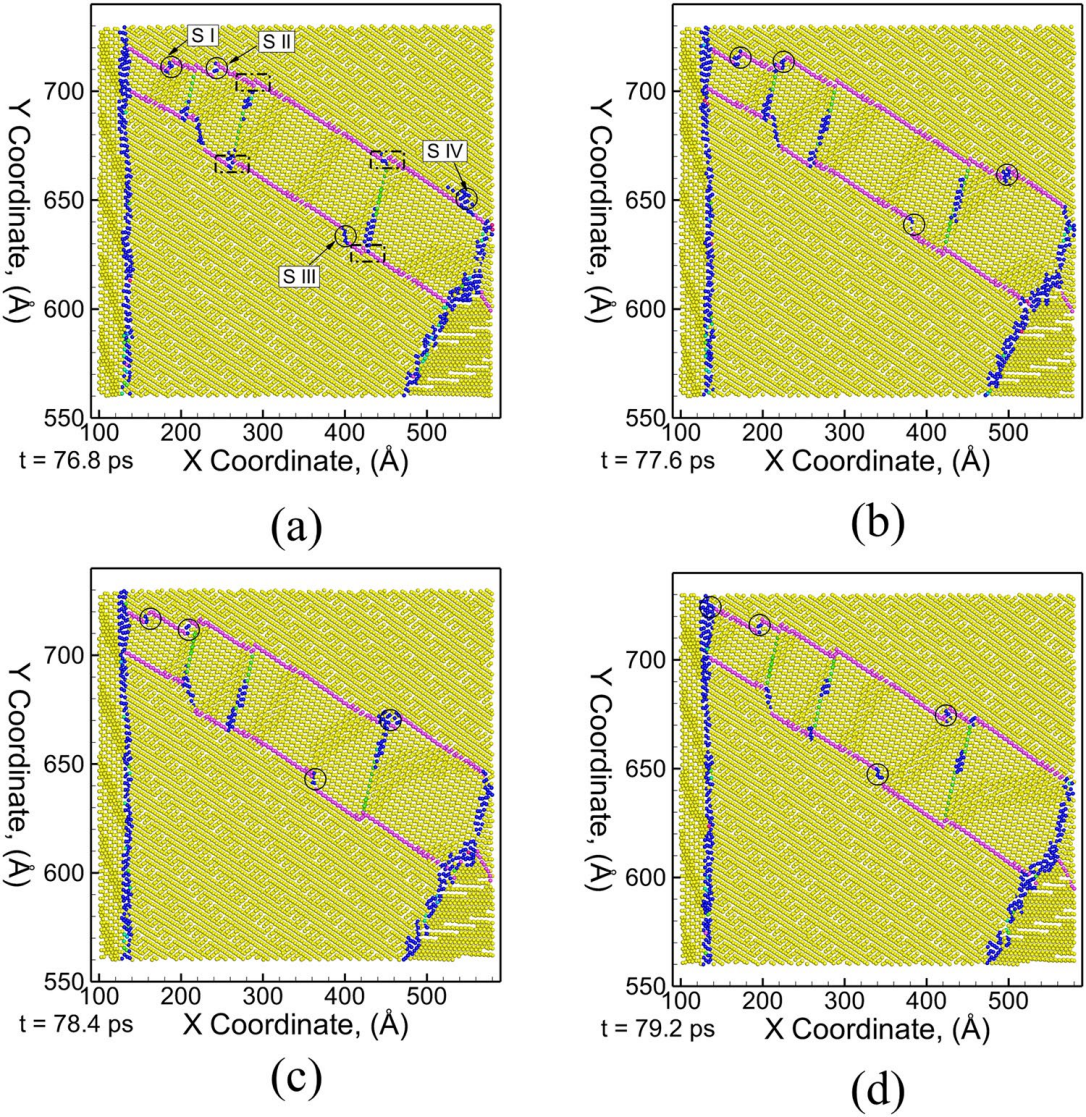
Microstructural snapshots depicting the growth of {$$10\bar{1}1$$} CT by movement of steps along the twin boundary during uniaxial tensile stress loading at a strain rate of 10^9^ s^−1^ at a time (strain) of (a) 76.8 ps (7.68%), (b) 77.6 ps (7.76%), (c) 78.4 ps (7.84%) and (d) 79.2 ps (7.92%). The atoms are colored using E-CNA method. Bulk HCP atoms are colored yellow, grain boundary/disordered atoms are colored dark blue, FCC atoms (basal stacking fault) are colored green, purple atoms represent {$${\rm{10}}\bar{1}1$$} CT boundary, light blue atoms represent {$${\rm{11}}\bar{2}1$$} TT boundary and red atoms represent surface/voids.
